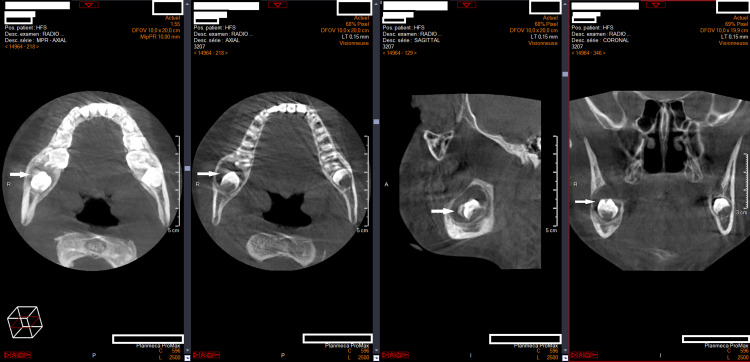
CBCT of a radiolucent lesion around the unerupted right mandibular second molar Axial, sagittal, and coronal CBCT views showing a well-circumscribed unilocular radiolucency with a thin sclerotic border surrounding the crown of the unerupted right mandibular second molar. White arrows indicate the radiolucent lesion enveloping the crown of the developing tooth germ. CBCT: cone-beam computed tomography
